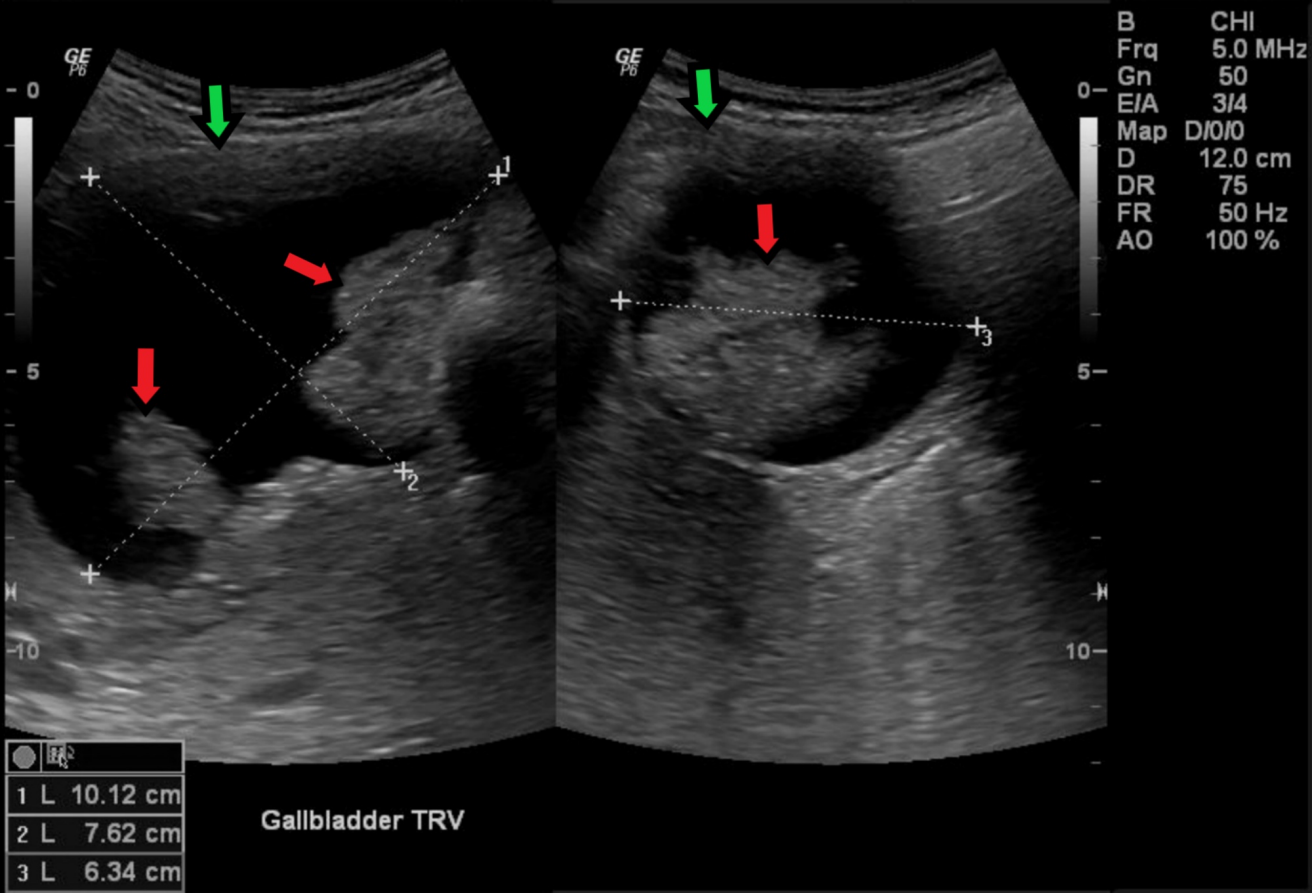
Transverse ultrasound of the gallbladder revealing multiple intraluminal masses (red arrows) and irregular wall thickening (green arrows). Gallbladder dimensions are given in the bottom left corner
